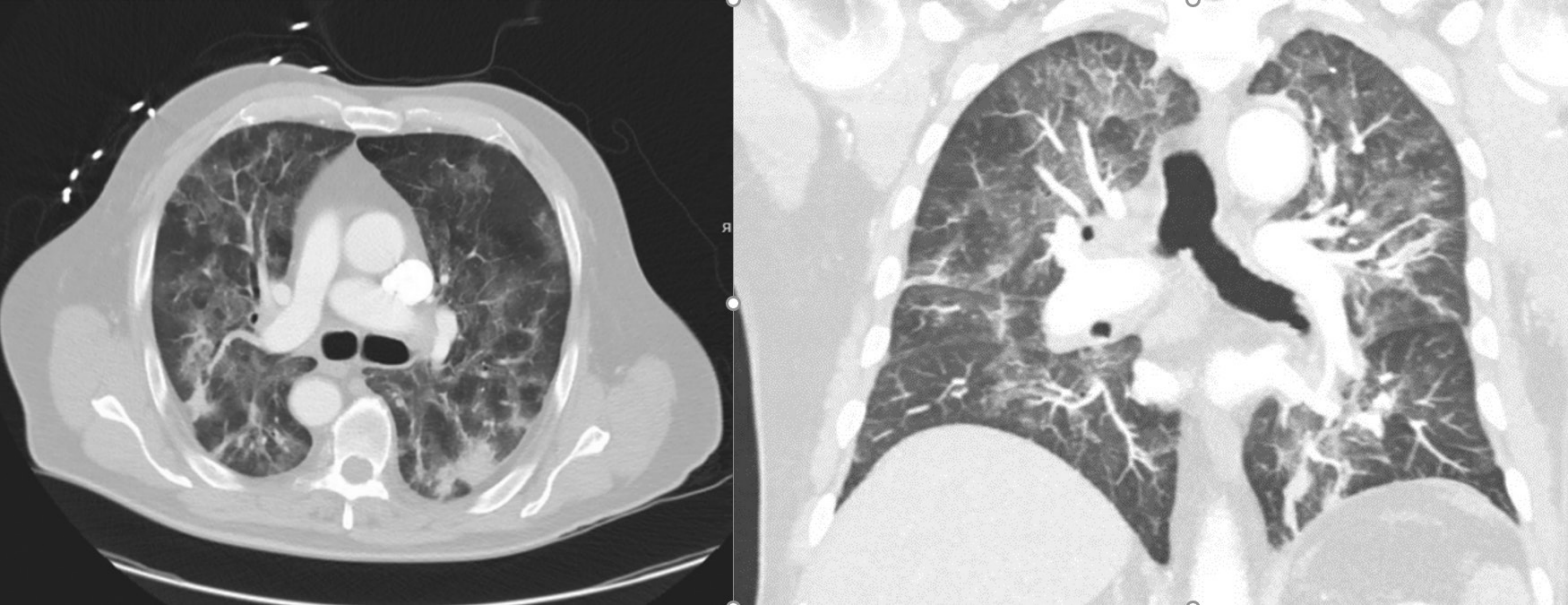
Representative slices of CT chest obtained on hospital Day 1 showed patchy ground‐glass opacity with areas of increased consolidation, especially in the left lower lobe.
